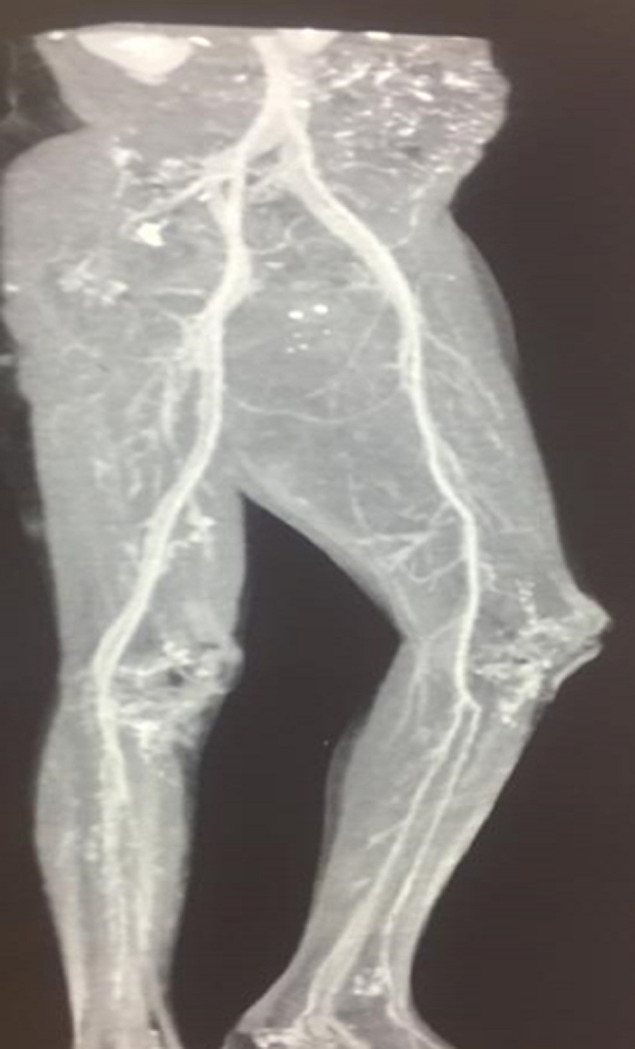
computed tomography angiogram of the distal extremities
